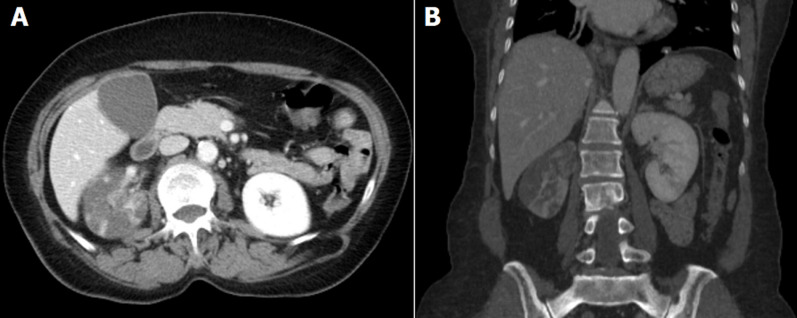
A) axial frame of the arterial phase of contrasted CT-scan in which is clearly visible the hypoperfusion of the right kidney compared to the left; B) coronal image of the abdomen in which is visible the hypoperfusion of the right kidney, especially in the upper pole
